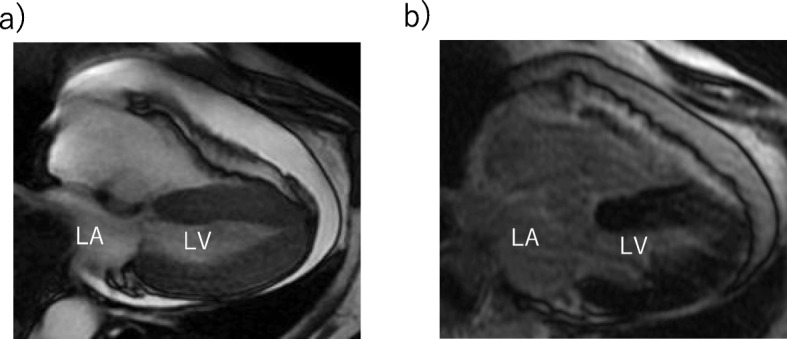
Magnetic resonance imaging (a: Fat suppressed T2 weighted image b: Delayed gadolinium enhancement image). Neither transmural late gadolinium enhancement nor cardiac edema were observed. (LA, Left Atrium; LV, Left Ventricle)
